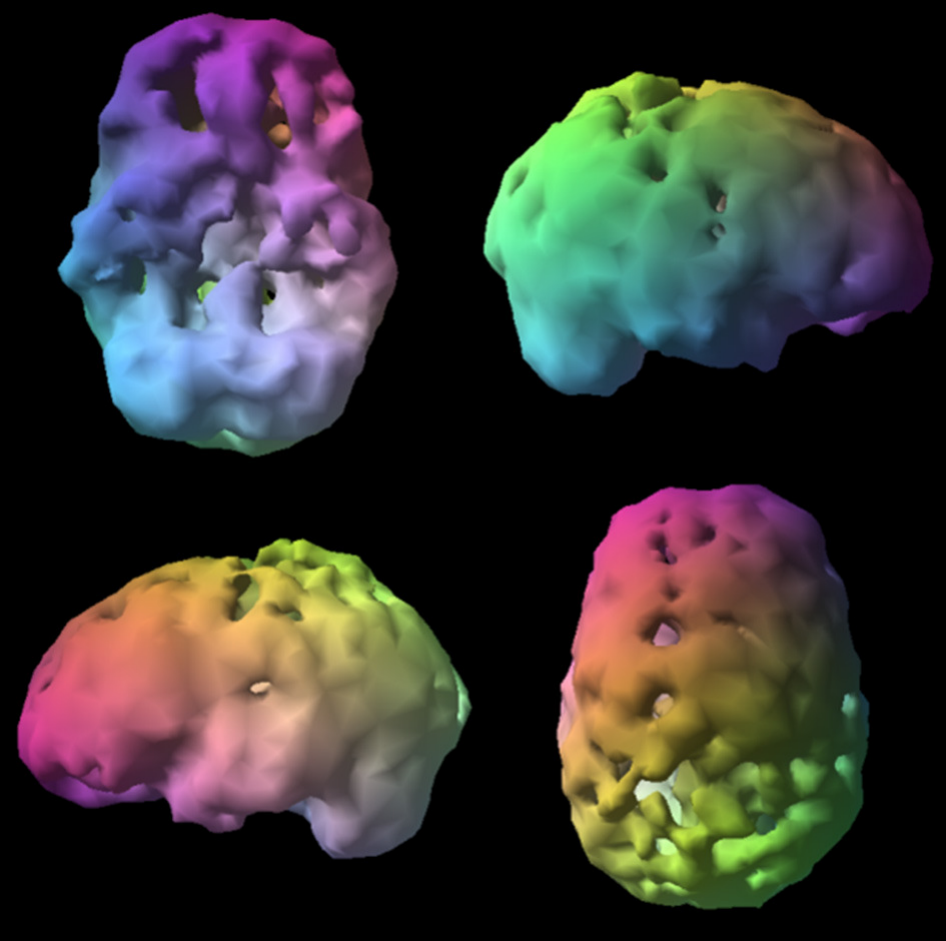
Toxic surface scan. Notice the scalloped, “Swiss cheese,” shriveled appearance indicating overall decreased perfusion. These are surface renderings of the SPECT information, looking at the top 45% of brain perfusion; anything below that level shows up as a hole or a dent. The holes do not mean no perfusion, they mean low perfusion, compared to a healthy dataset.
